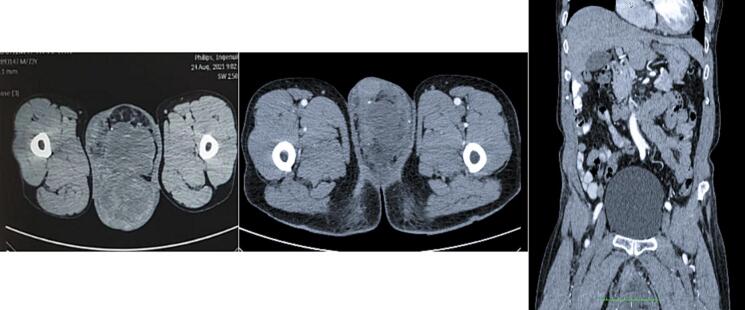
Abdominal and Pelvic CT-Scan showed an enhanced contrast effect in the lesions.
